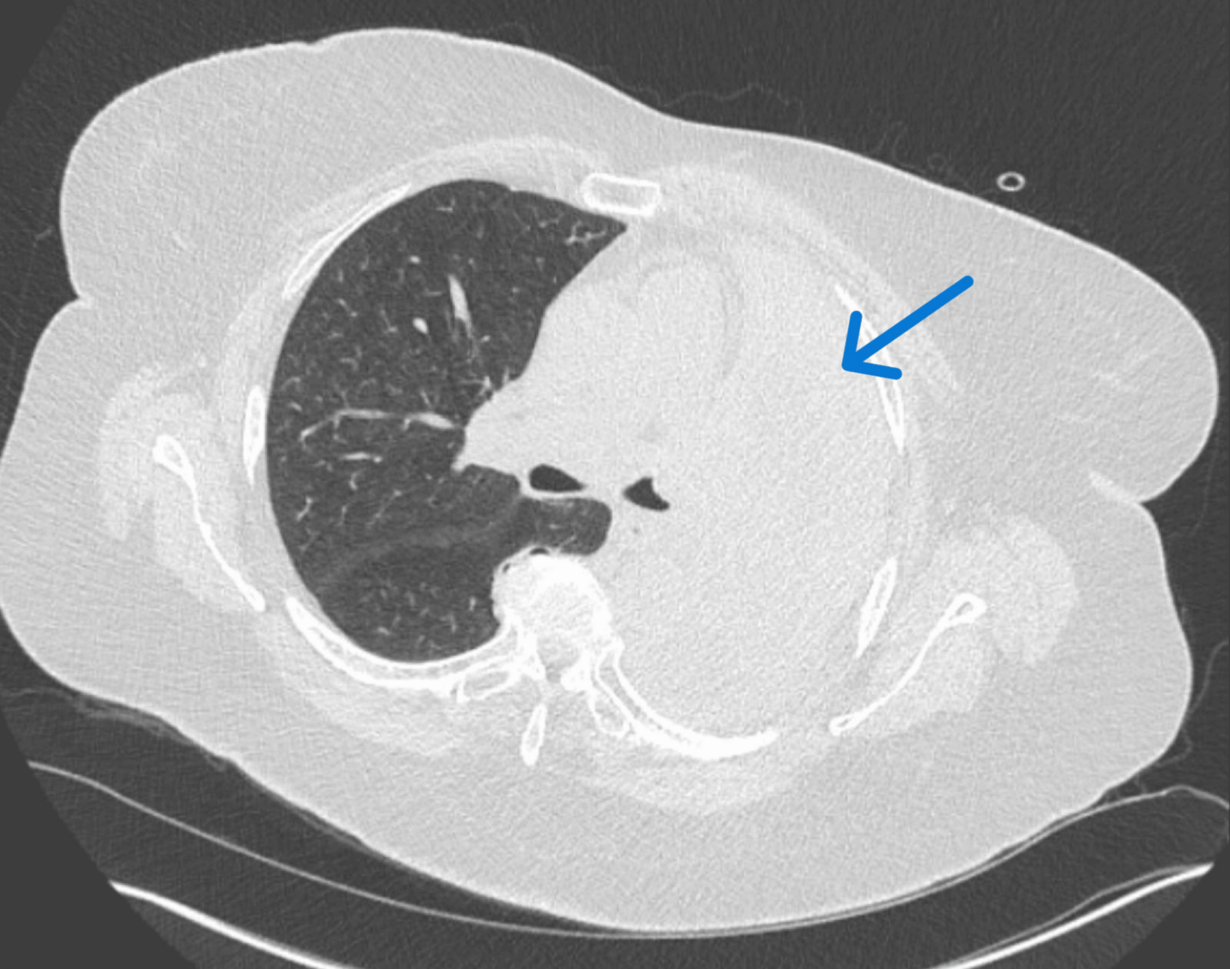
CT Chest Two Weeks Prior to Hospitalization Stable left bronchus occlusion secondary to longstanding squamous cell lung carcinoma (blue arrow).
